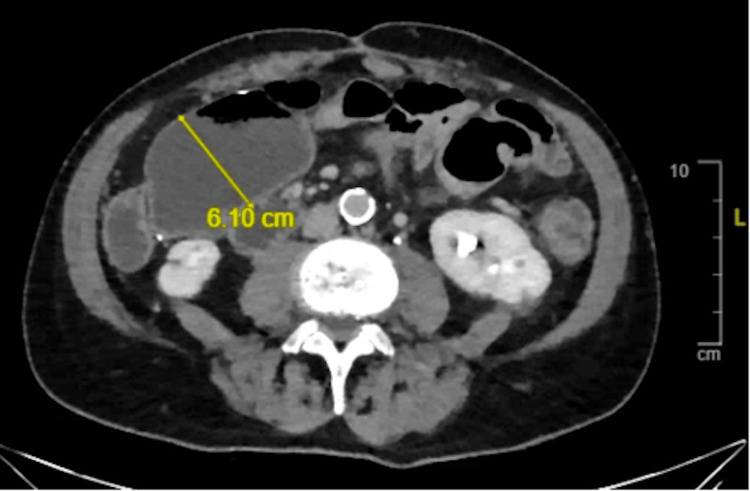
CT showing circumferential transmural wall thickening of the distal ileum with skip lesions
